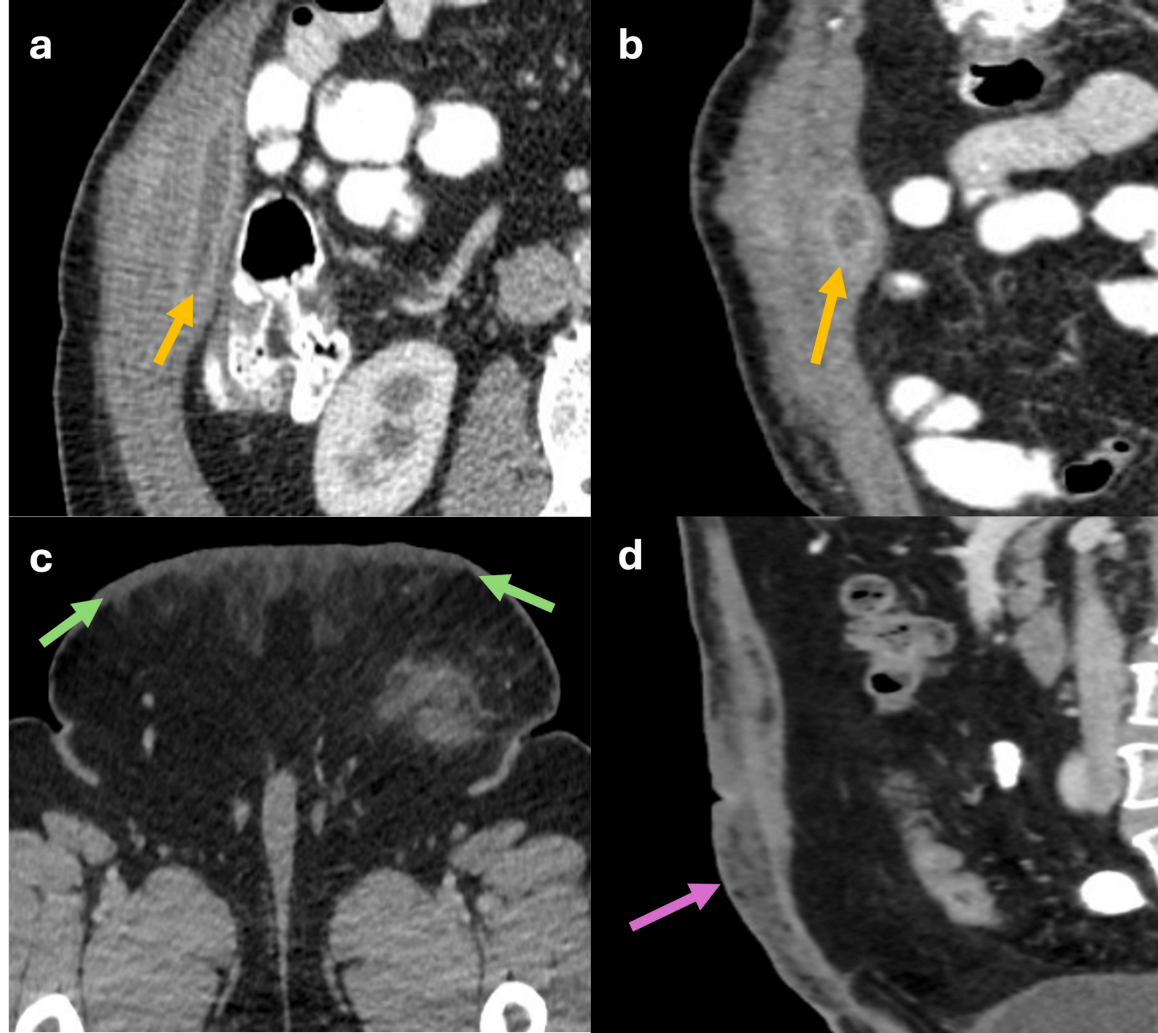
Axial, coronal, and sagittal abdominal CT scan showing an intramuscular abscess and cellulitis (a, b) Axial and coronal CT shows an intramuscular rim enhancing fluid collection inside the right transversus muscle consistent with an abscess (yellow arrows). (c, d) Axial and sagittal CT scan showing thickening of the skin and subcutaneous tissue (green arrows) along with fat stranding (pink arrow), consistent with cellulitis. The figure was created by the authors.
